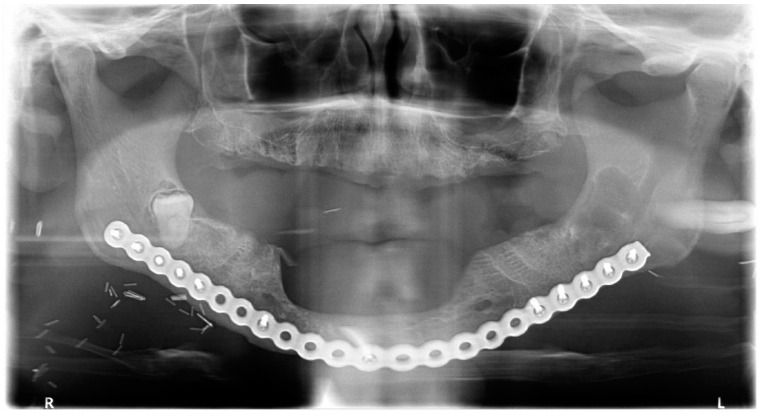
CBCT X-ray of case 4 at one year follow-up.
